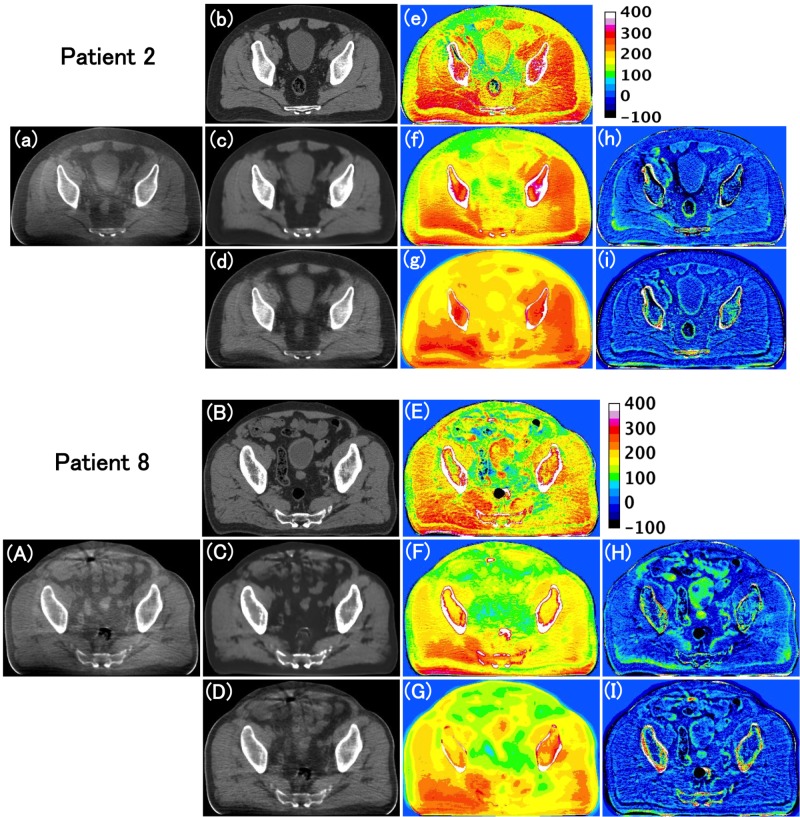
Comparison among original CBCT, pCTr, i-CBCT and enhanced CBCT for patient 2 and 8. (a), (b), (c) and (d) are original CBCT, pCT_r_, i-CBCT and enhanced CBCT, respectively; (e) pCT_r_ minus original CBCT; (f) i-CBCT minus original CBCT; (g) enhanced CBCT minus original CBCT; (h) pCT_r_ minus i-CBCT; (i) pCT_r_ minus enhanced CBCT for patient 2. (A) to (I) are for patient 8. original CBCT: Original cone beam computed tomography; pCTr; Registered planning computed tomography; i-CBCT: Improved cone beam computed tomography; enhanced-CBCT: Enhanced cone beam computed tomography.
